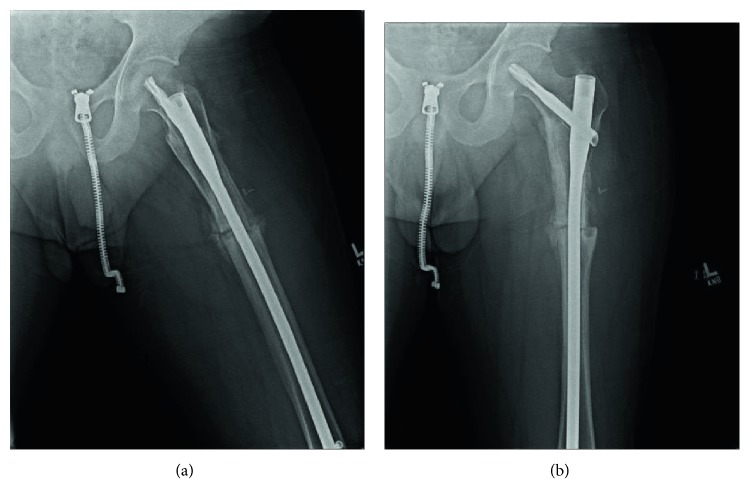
(a and b) Postoperative radiographs showing a healing fracture with abundant callus along the cortex.
